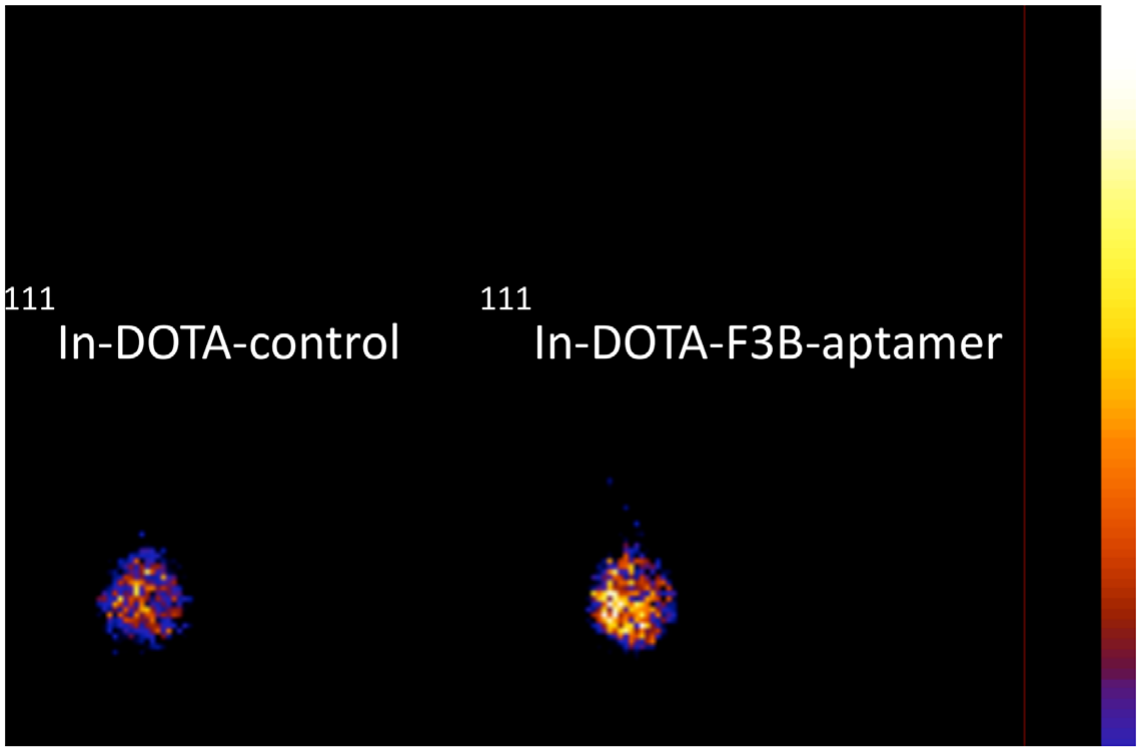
Ex vivo scintigraphic planar image of posterior paw at 1h after i.v. injection of ^111^In-DOTA-F3B-aptamer and 111In-DOTA-control-aptamer.
